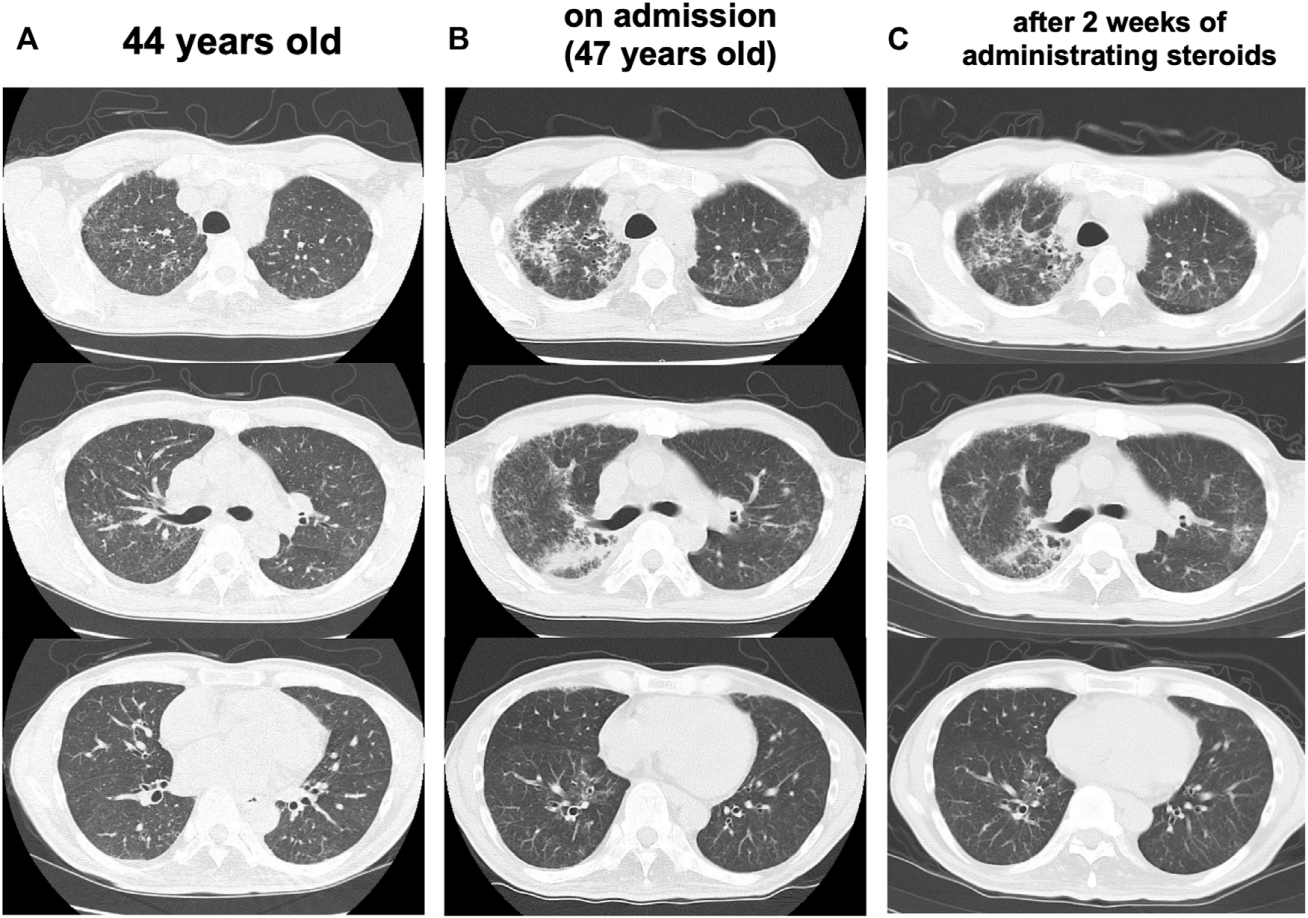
Progress of lung CT scans (A) At 44 years old (B) On admission (47 years old), the interstitial lung shadows worsened (C) 2 weeks after administrating corticosteroids, interstitial shadows were improved.
